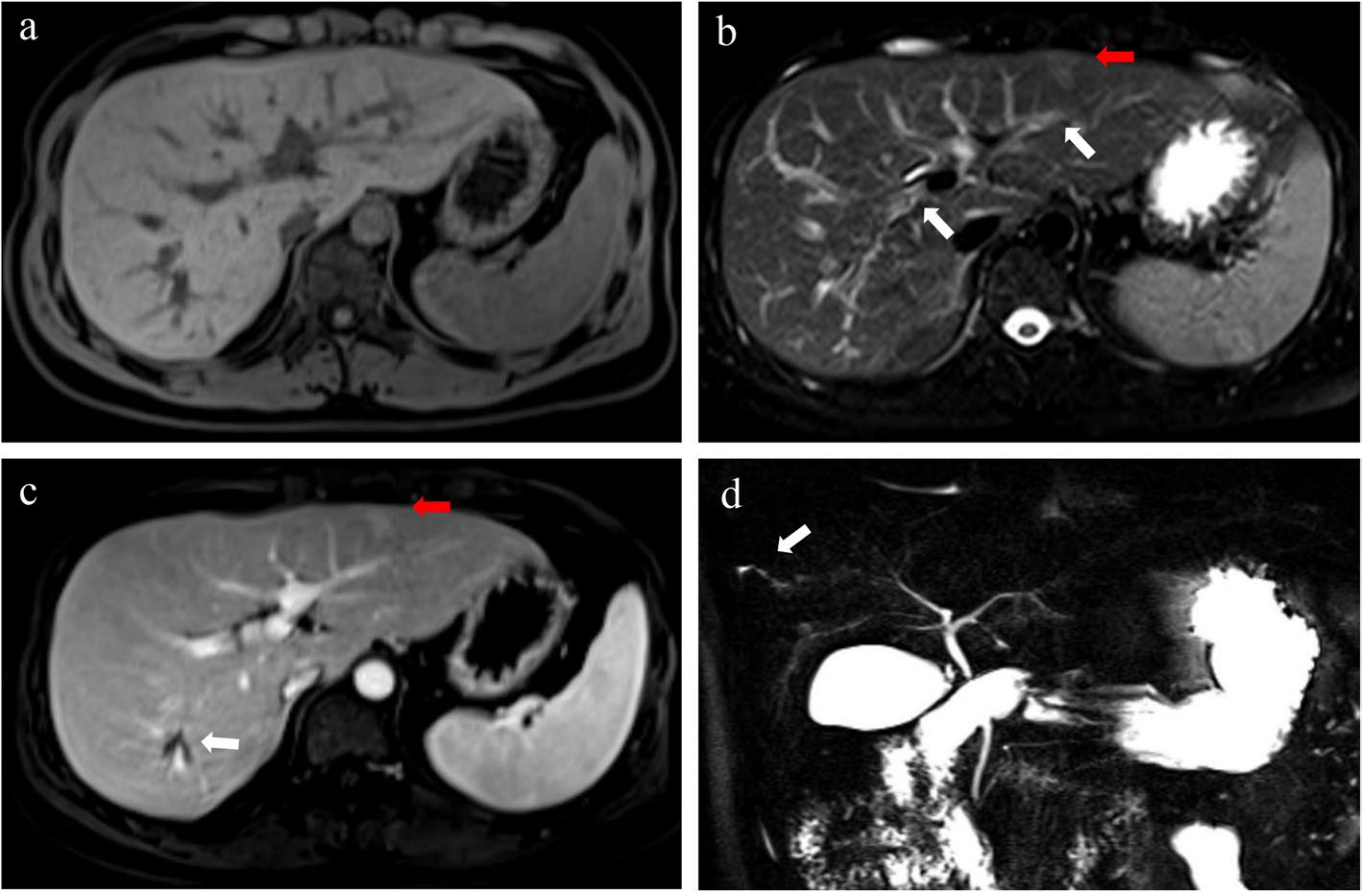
Contrast-enhanced axial MRI scans in a 25-year-old female with PBC (stage I–II, Ludwig staging system) (a–d). The liver has a regular contour without nodular changes of the surface on the T1WI image (a). The T2WI image shows periportal hyperintensity (white arrows), and small patchy heterogeneity in segment II (red arrow) (b). The PVP image shows mild heterogeneous enhancement of the liver parenchyma (red arrow) with dilated small bile ducts in segment VII (white arrow) (c). The MRCP image shows segmental irregular dilatation of the small bile ducts in the right lobe of the liver (arrow) (d)
